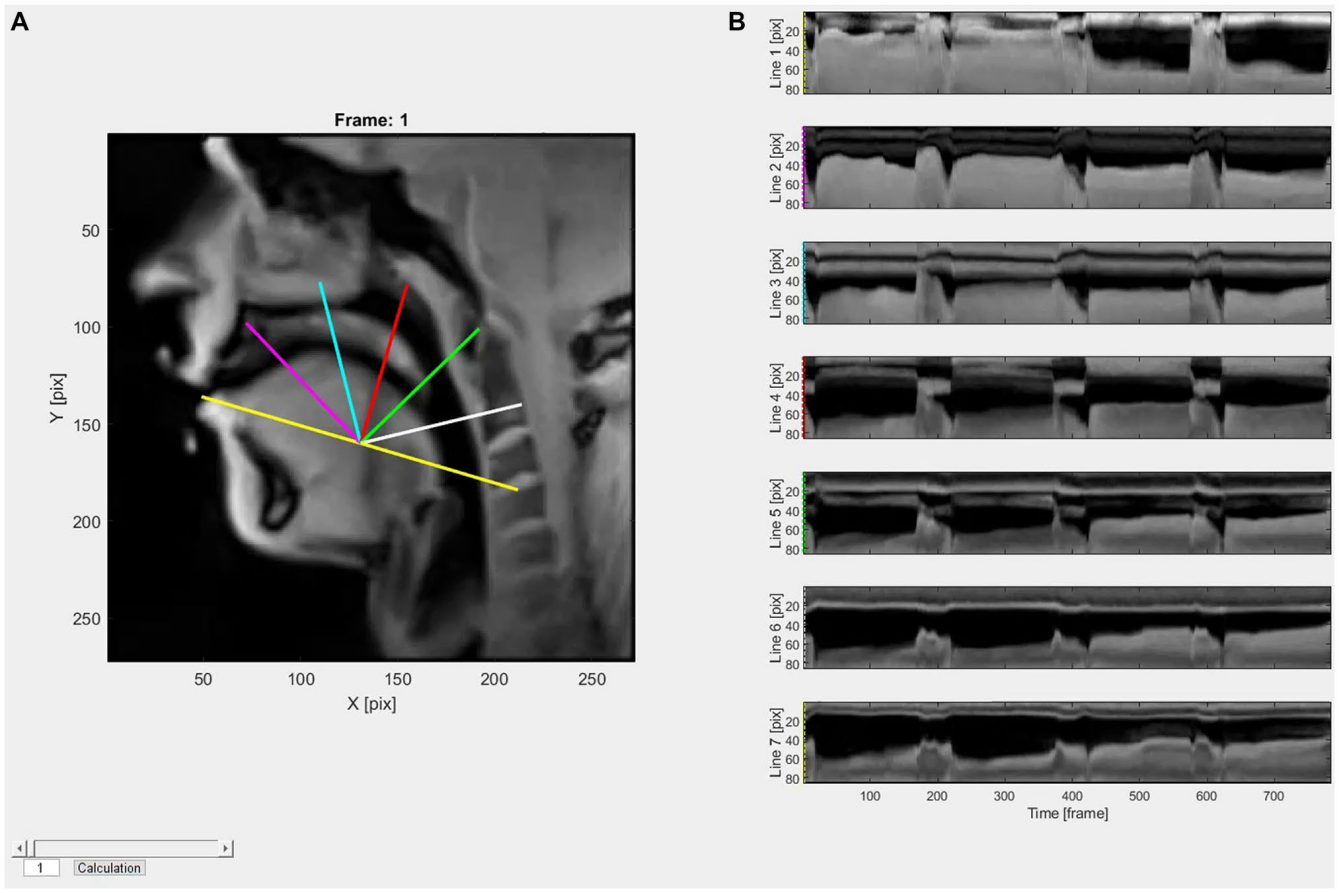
The localization of the 7 PLs is shown in panel A. The different PLs are visualized in different colors and are projected over the MRI images with the software MATLAB. Time-dependent changes in tongue edge position (pixels) along each line are presented in panel B. The colors at the beginning of each graph in panel B are linked to the PL colors in panel A.
